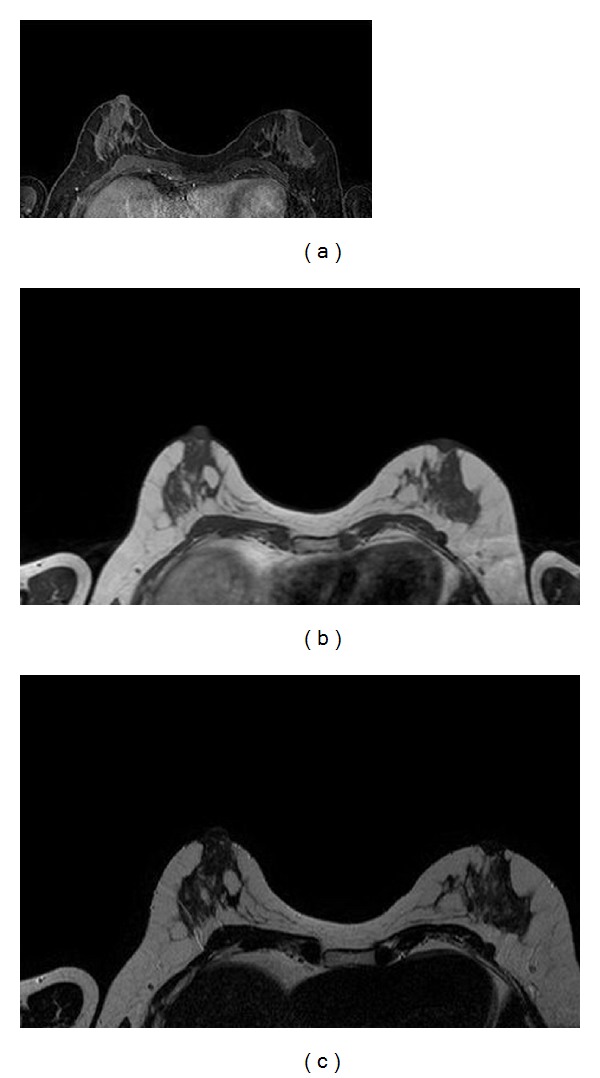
Different MRI (T1 with fat suppression, T1, T2) with skin intensity level similar to dense tissue intensity level.
